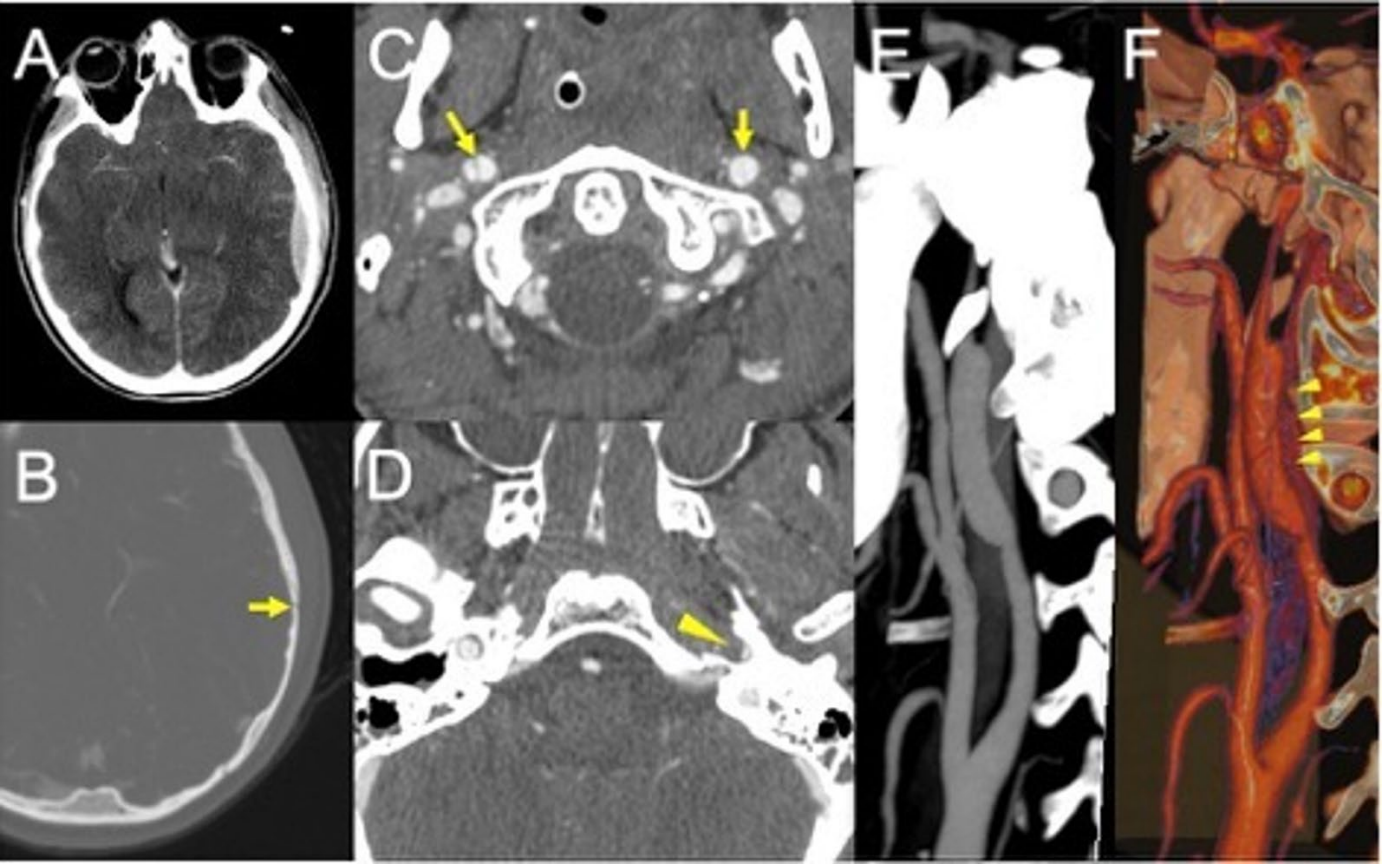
Post-traumatic bilateral internal carotid dissection and left carotid fusiform dilatation in an adolescent patient following a motor vehicle accident. CT scan shows a parieto-temporal epidural hemorrhage (A) in association with a skull fracture at the same level (B, arrow). CTA demonstrates a bilateral double lumen sign (C, arrows), consistent with the presence of intimal flap and a distinct narrowing of the left vertical petrous carotid (D, arrowhead). Maximum intensity projection (E) and 3D volume rendering (F, arrowheads) show the presence of a dilatation of the left carotid artery (consistent with a pseudoaneurysm due to a tear of the intima and hemorrhage into media and adventitia layers)
